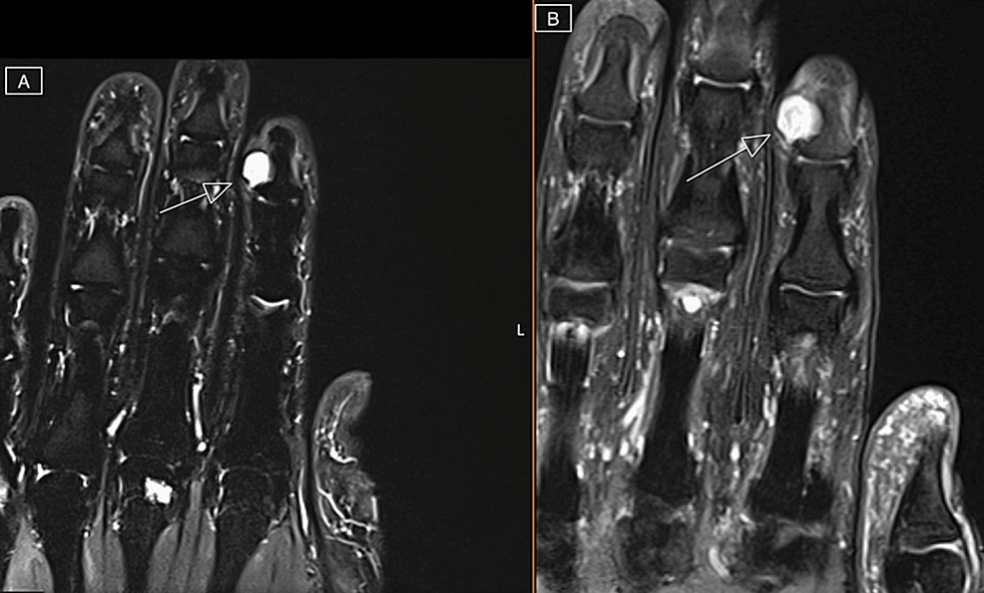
Coronal view of initial MRI scan depicting a parosteal lesion, likely to be a glomus tumor of the right index finger. (A) Post-contrast. (B) T2-weighted fat suppressed.
